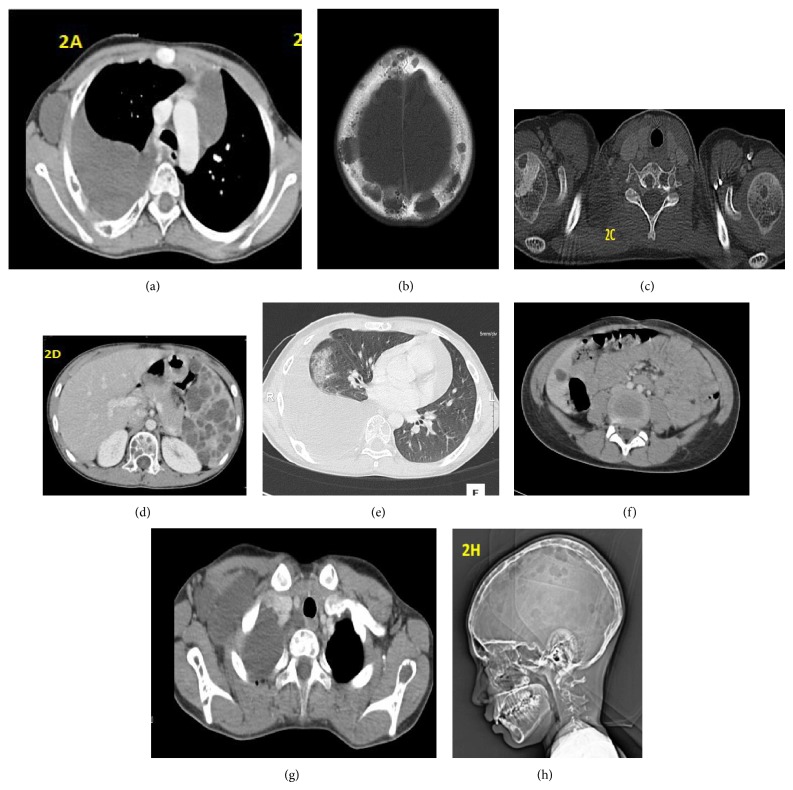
(a) CT scan chest (axial section) showing right pleural effusion, left mediastinal effusion, right chest wall hypodense lesion, and osteolytic lesions in ribs on right side. (b) CT scan skull (bony window) showing multiple osteolytic lesions without marginal sclerosis. (c) CT scan through shoulders (bony window) showing osteolytic lesions in head of each humerus without marginal sclerosis and vertebral body lesion with marginal sclerosis. (d) CT scan abdomen showing multiple cystic lesions in spleen with splenomegaly and osteolytic lesions in vertebra. (e) CT scan thorax (pulmonary window) showing pulmonary lymphangioma appearing as nonhomogenous consolidation with few small cystic hypodense shadows laterally with right pleural effusion. (f) CT scan abdomen showing hypodense lesion in liver. (g) CT scan of upper chest showing hypodense lesion in right axilla and pleural effusion with hypodense lesion in left scapula. (h) Skull topography showing multiple osteolytic “punched-out” lesions.
